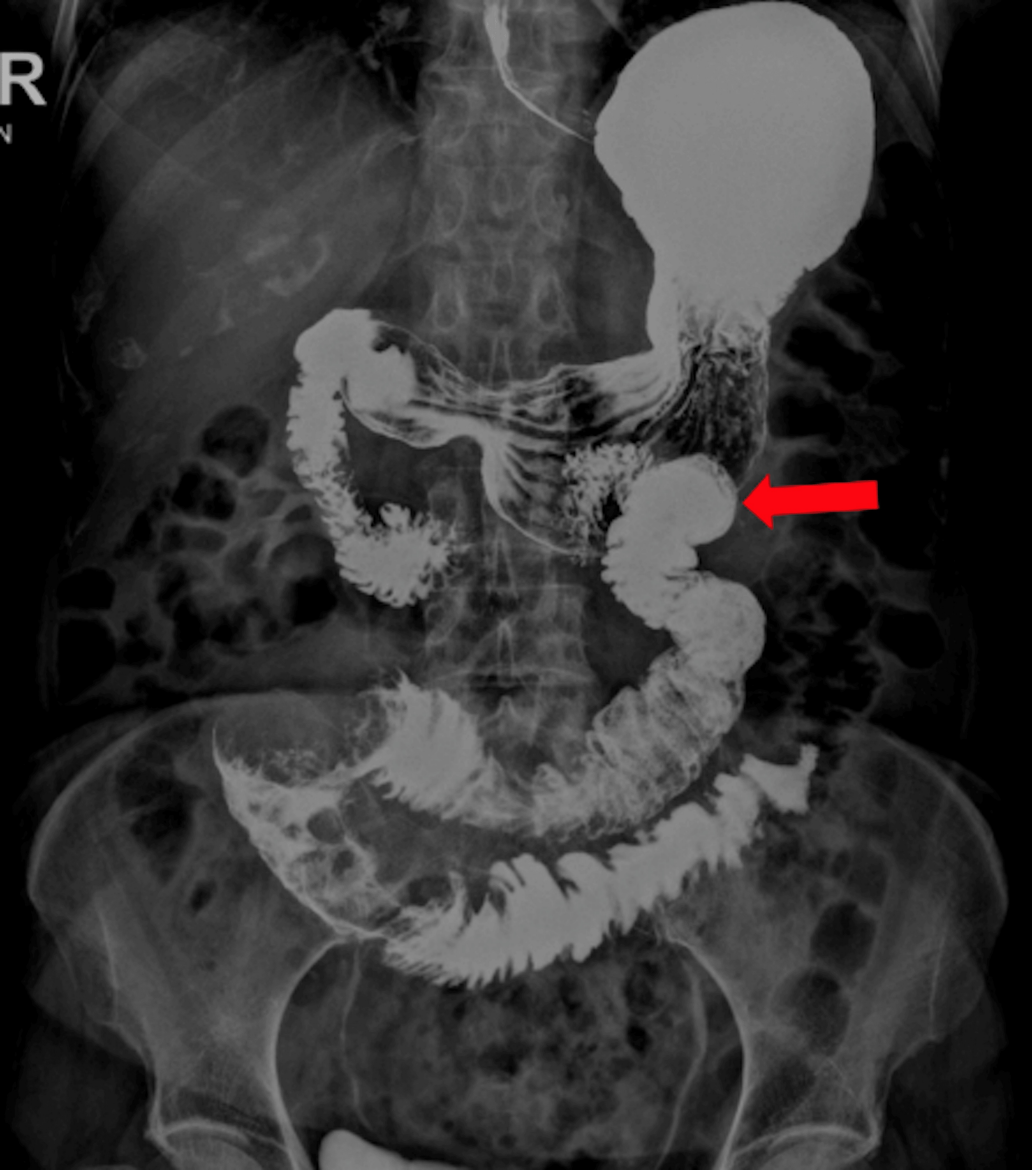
X-ray of the abdomen (anterior-posterior supine and erect views), barium meal, and follow-through showing filling defects of 5.8 cm and 5.2 cm seen at the proximal jejunal loop (red arrow).
